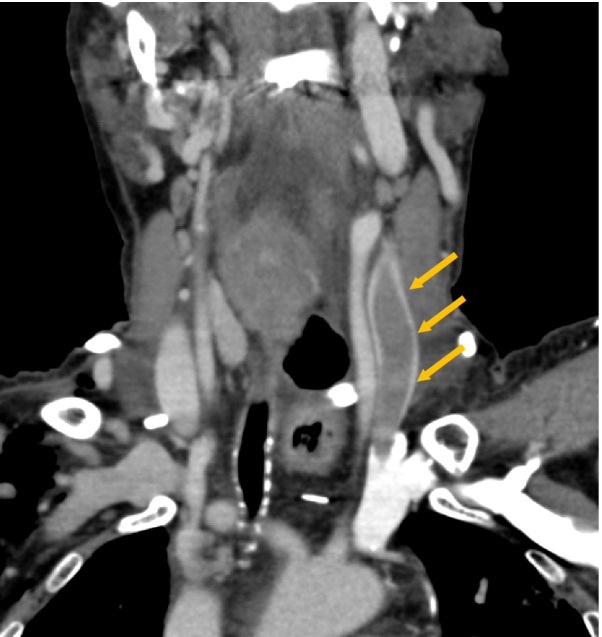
Upper extremity deep vein thrombosis in the left internal jugular vein after esophagectomy with retrosternal reconstruction. Contrast-enhanced CT on the fourth postoperative day and the open arrows indicate an intraluminal filling defect in the left internal jugular vein
